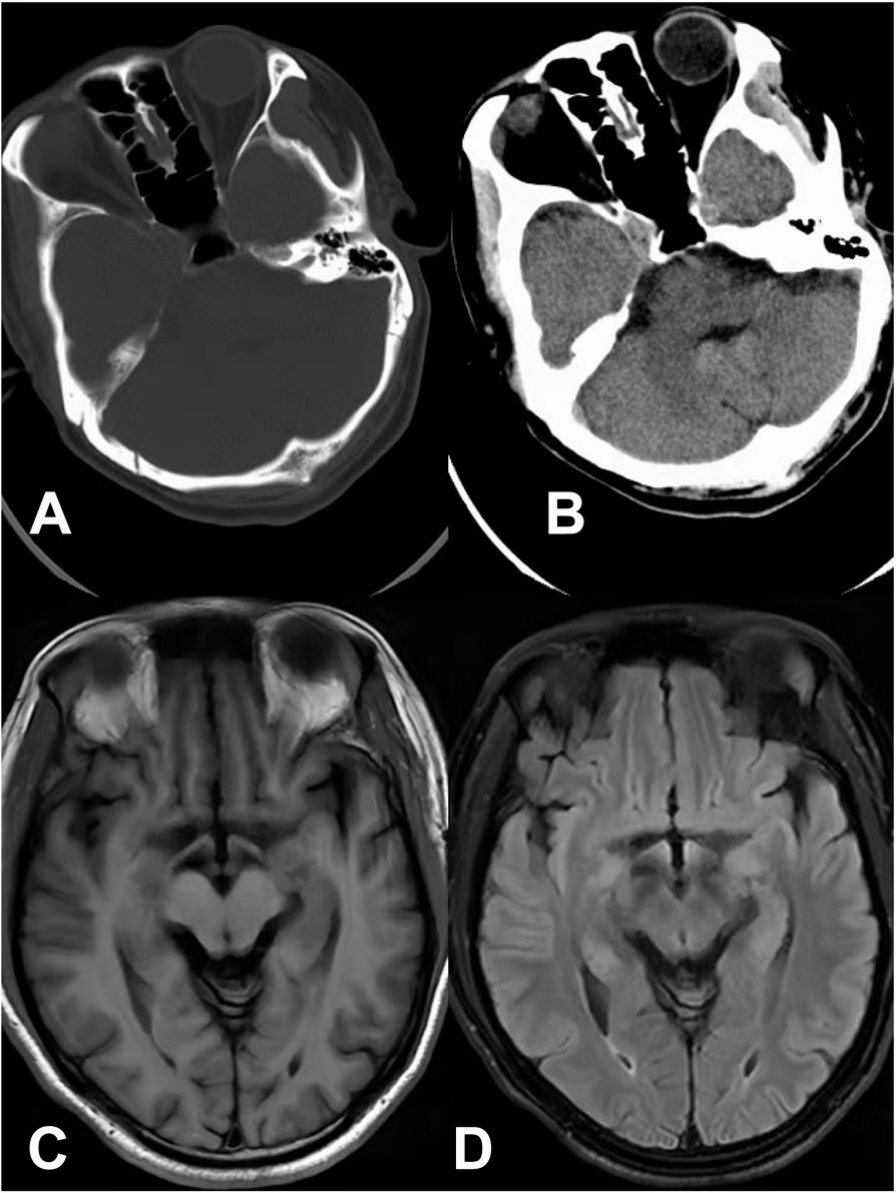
A and B show the head computed tomography scan of the patient; C and D show the head magnetic resonance imaging scan of the patient
